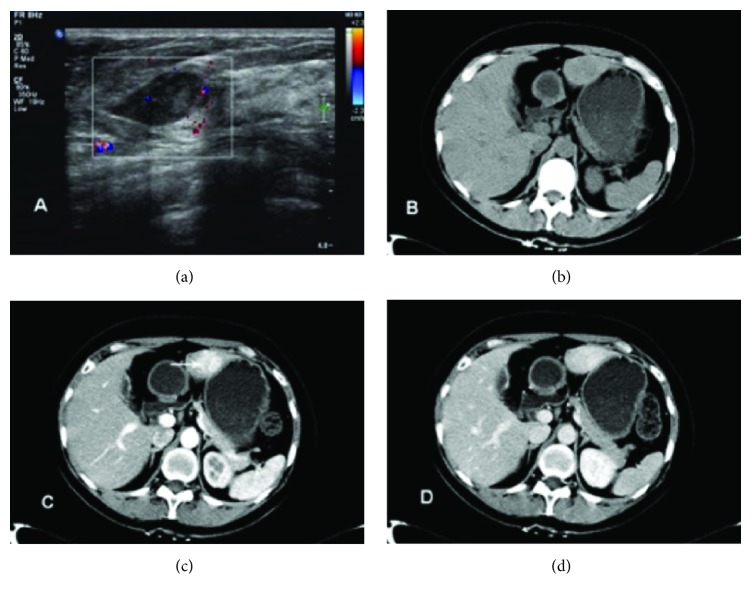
Abdominal ultrasonography (USG) and CT findings: (a) USG shows a 3 × 3cm slightly hypoisoechoic hepatic lesion in left lateral lobe with slightly unclear borders and blood flow signal; (b) plain CT shows that the lesion is lobulated, homogeneous, and isointense compared to surrounding liver parenchyma; (c) enhanced CT shows that hypervascular lesion appears hyperdense with a slightly hypodense central scar in the arterial phase (arrows); (d) in the portal venous phase the lesion is isodense without signs of central scar.
